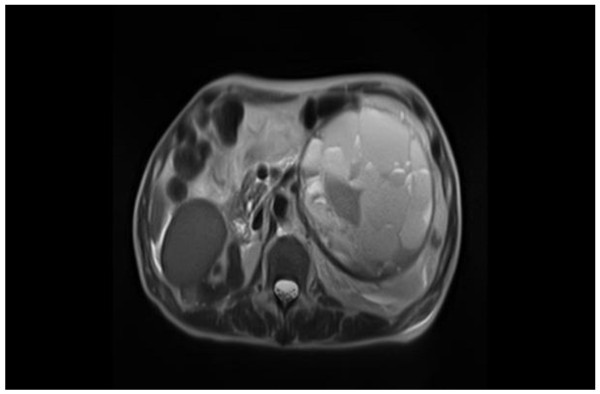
Abdominal MRI. The T2-weighted images show high signal intensity in a major portion of the mass. Heterogeneous signal intensity with hyperintense and hypointense areas is also observed.
